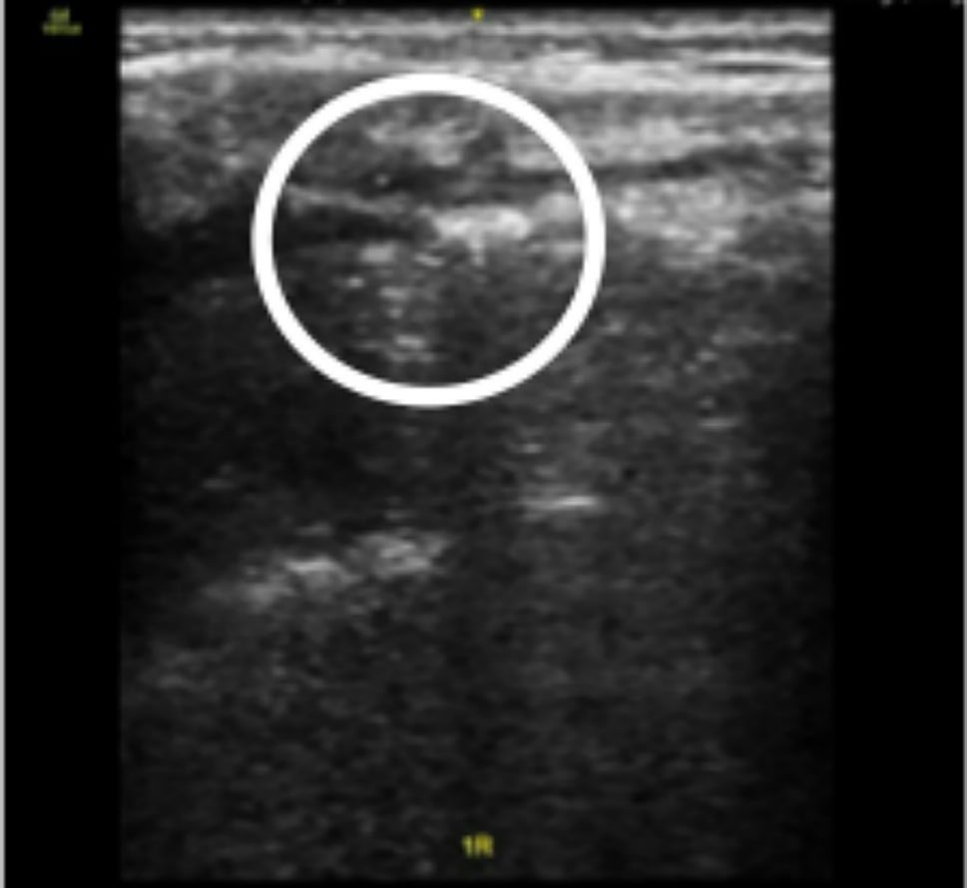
Ultrasound image of the lung-point (circled) of a patient presenting with pneumothorax
